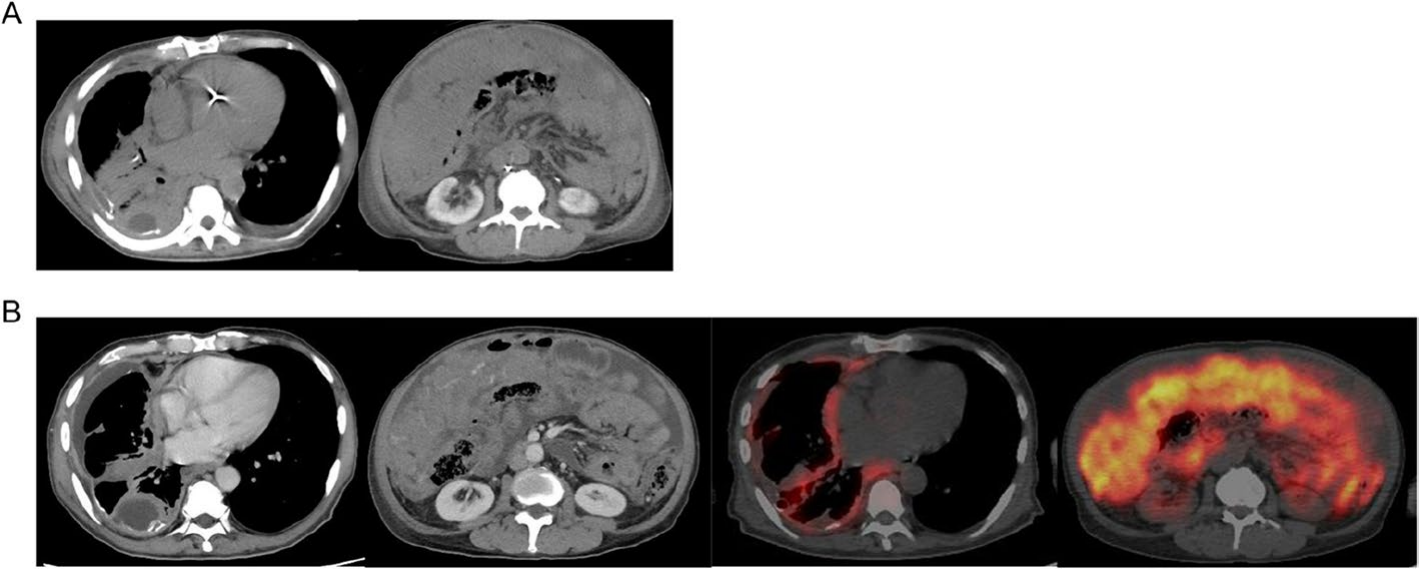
Baseline and post-treatment imaging. a CT (computed tomography) showing right pleural thickening, peritoneal dissemination, tumor-like soft tissue shadows, and ascites at the time of presentation to the emergency department. b Baseline CT and PET showing right pleural thickening, peritoneal dissemination, tumor-like soft tissue shadows, and ascites at baseline
